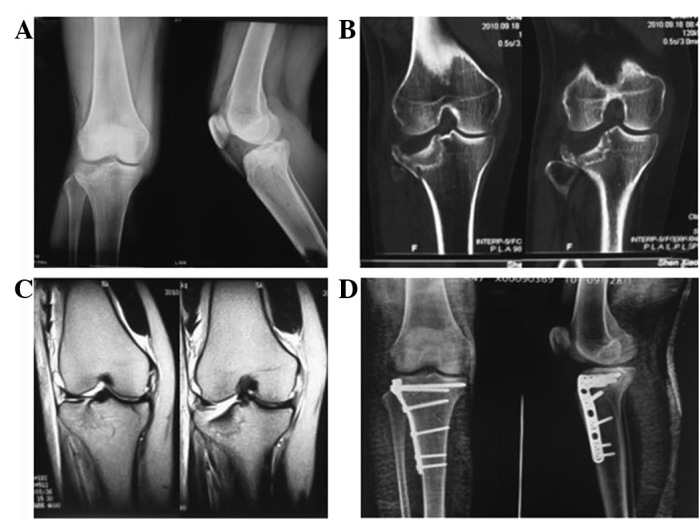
Female, 38 years old, with collapse fractures of the right tibial platform. (A) Preoperative X-ray. (B) Preoperative computed tomography (CT) scan showing the lateral central collapse of the right tibial platform of ~2 cm. (C) Preoperative magnetic resonance imaging (MRI) scan showing the lateral tibial plateau fracture and the complete disruption of the medial collateral ligment. (D) Postoperative X-ray images showing the anatomical reduction of the right lateral tibial plateau fracture and the flat joint surface.
